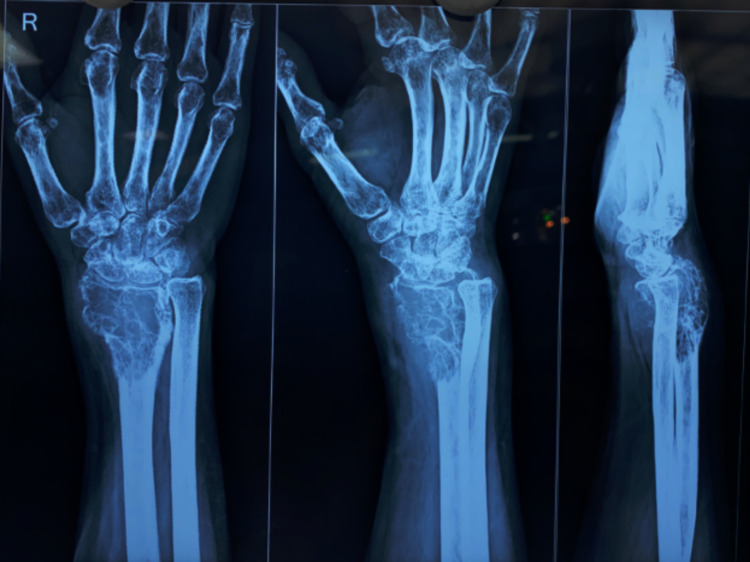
Pre-operative radiograph of right wrist showing locally aggressive giant cell tumor (GCT) of the right distal radius.
